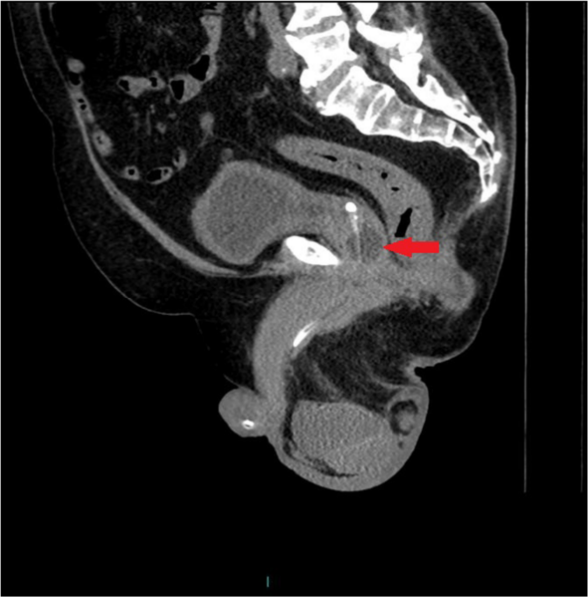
Sagittal section of CT of pelvis: the balloon of Foley catheter (arrow) is located in dilated prostato-membranous urethra.
